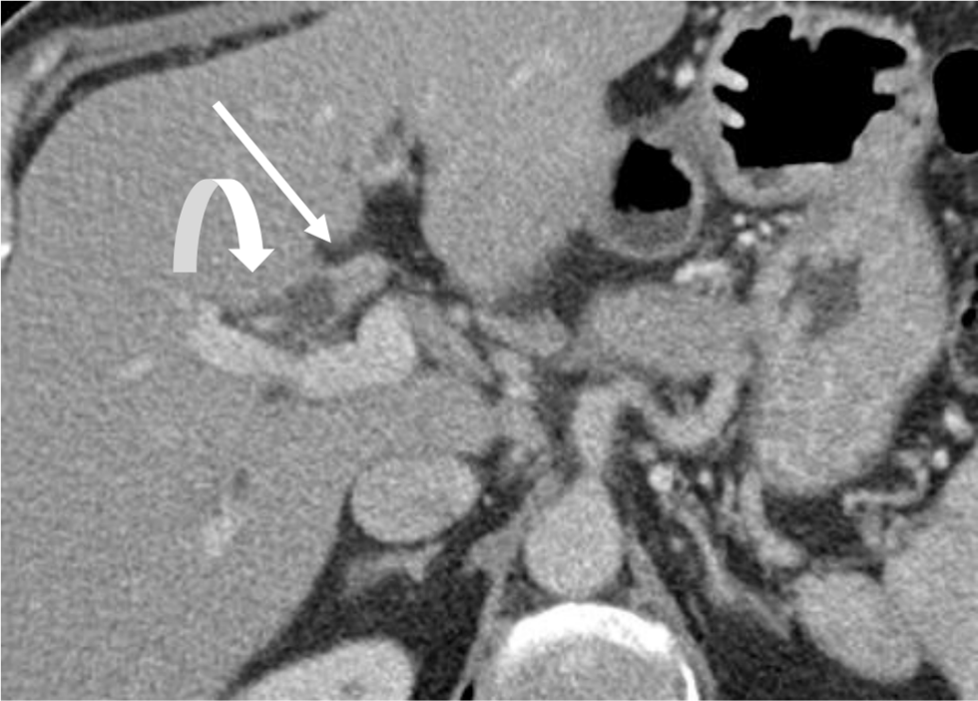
Large Duct Cholangiocarcinoma. Axial CT images with contrast demonstrate a periductal infiltrative mass (arrow), with wall thickening and enhancement of the common hepatic duct with dilated upstream bile ducts (curved arrow)
